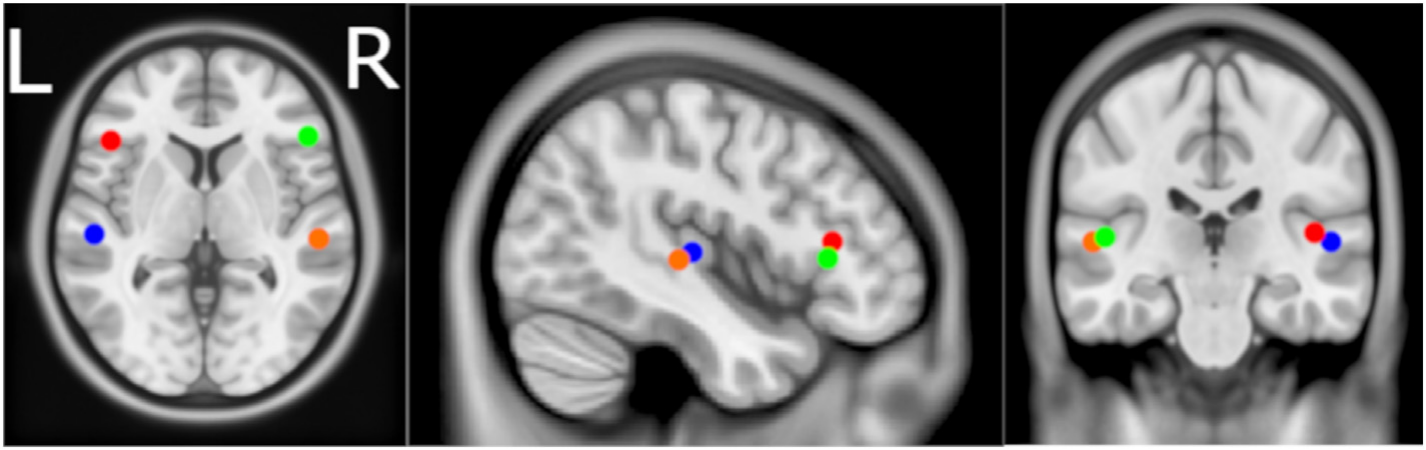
Location of dipoles modelled by dipole fitting. Centroids of the left (blue) and right (orange) superior temporal gyri and left (red) and right (green) inferior frontal pars triangularis were used to seed dipoles for dipole fitting. Axial MRI view is from above (L-Left, R-Right).
